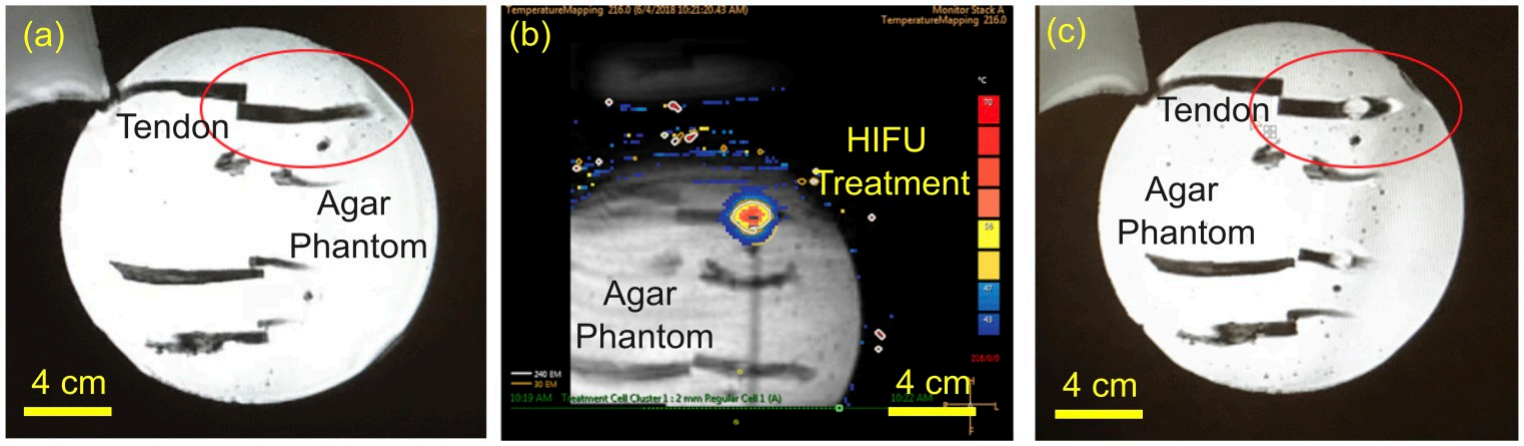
Coronal views of MRgFUS targeted tendon (a) T2-MRI prior to MRgFUS, (b) during MR thermometry, and (c) following MRgFUS ablation showing hyperintense area.
